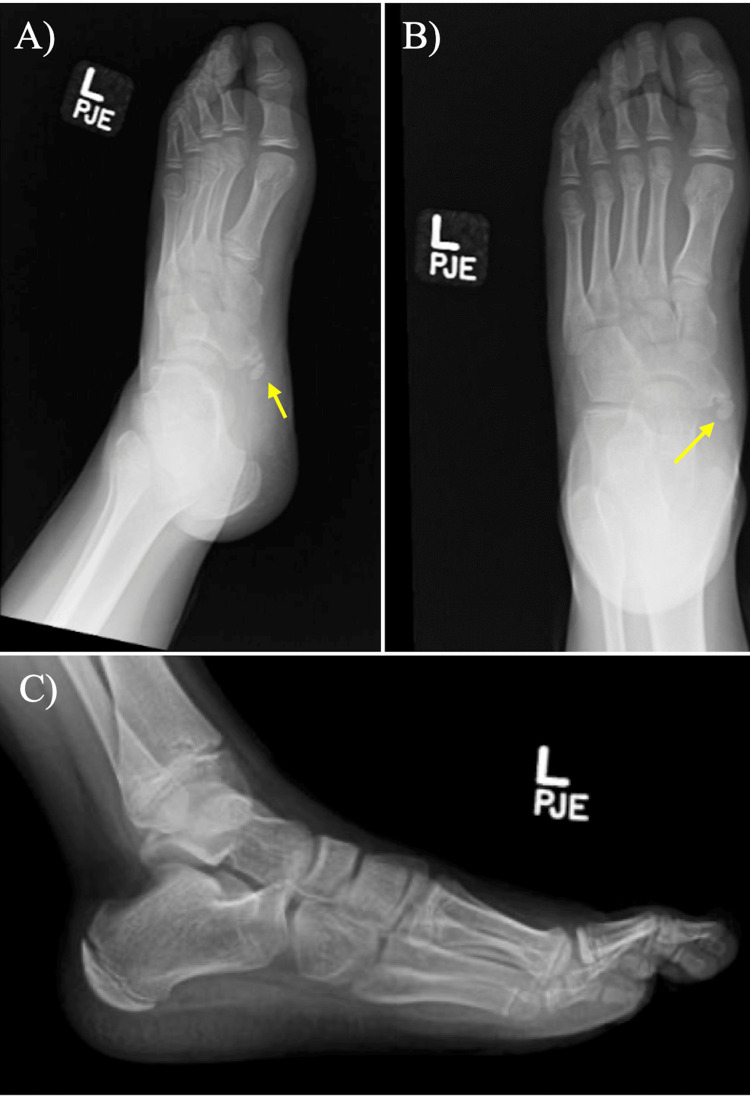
Repeat preoperative foot and ankle radiographs. A) Oblique view X-ray of the left foot, six months post-injury, demonstrating two distinct ossification centers (yellow arrow); B) Anterior-posterior (AP) view of the left foot, six months post-injury, demonstrating two distinct ossification centers (yellow arrow); C) Lateral view X-ray of the left foot. Ossicles are occluded by the remainder of the foot.
